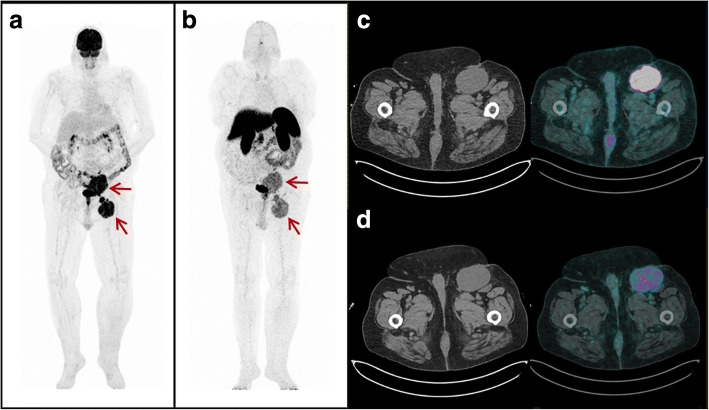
PET/CT images performed after chemotherapy in patient #8, who presented with UPMCC diagnosed by left inguinal lymph node biopsy. Maximum intensity projection (MIP) ^18^F-FDG PET/CT (a) and ^68^Ga-somatostatin analog (b) PET/CT images concordantly showed abnormal tracer uptake in multiple left iliac and inguinal lymph nodes (red arrows). Transaxial ^18^F-FDG (c), and ^68^Ga-somatostatin analog (d) PET/CT images concordantly showed abnormal tracer uptake (higher with ^18^F-FDG) in enlarged pathological left inguinal lymph node
